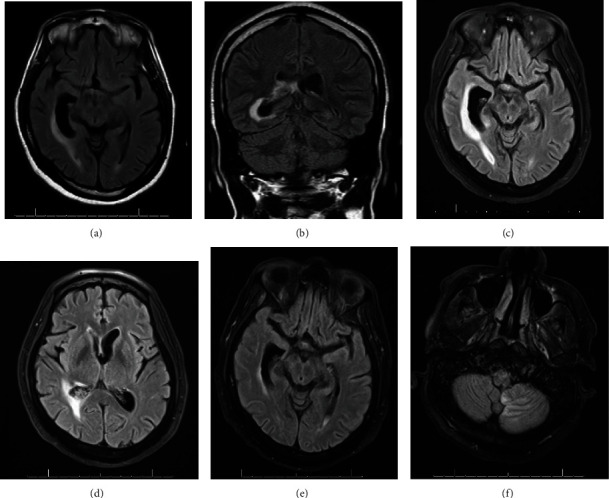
(a, b) (MRI brain T2/FLAIR): panels (a) [axial] and (b) [coronal] with hyperintense signal in the periventricular white matter along the right occipital horn with asymmetric dilation of the temporal horn of the right lateral ventricle. (c, d) (MRI brain T2 qTSE [axial]): panel (c) is showing asymmetric enhancement of the choroid plexus within the right lateral ventricle, mild ependymal enhancement, and foci of high diffusion signal within the lateral ventricles bilaterally, which are compatible with ventriculitis. Panel (d) additionally with new high diffusion signal along the occipital horn of the left lateral ventricle and new acute ischemic infarction of the lower cervicomedullary junction (not shown). (e, f) (MRI T2/FLAIR [axial]): panel (e) shows the right ventricle asymmetrically enlarged relative to the left with ongoing but decreased adjacent hyperintensity. Focal diffusion restriction within the left lateral ventricle was more prominent and was thought to represent evolving abscess formation of the choroid plexus in the setting of ventriculitis, and layering pus visualized in the posterior horn left lateral ventricle. Panel (f) shows a new area of diffusion restriction within the left inferior vermis and left inferior cerebellar hemisphere with associated cytotoxic edema concerning for acute/subacute infarct.
